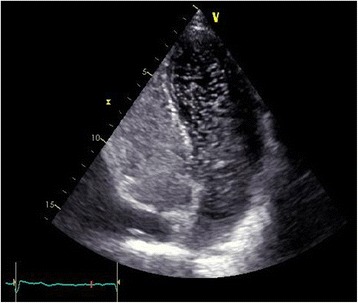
Contrast-enhanced transthoracic echocardiography. Passage of microbubbles to the left atrium during the first three beats after right atrial opacification
